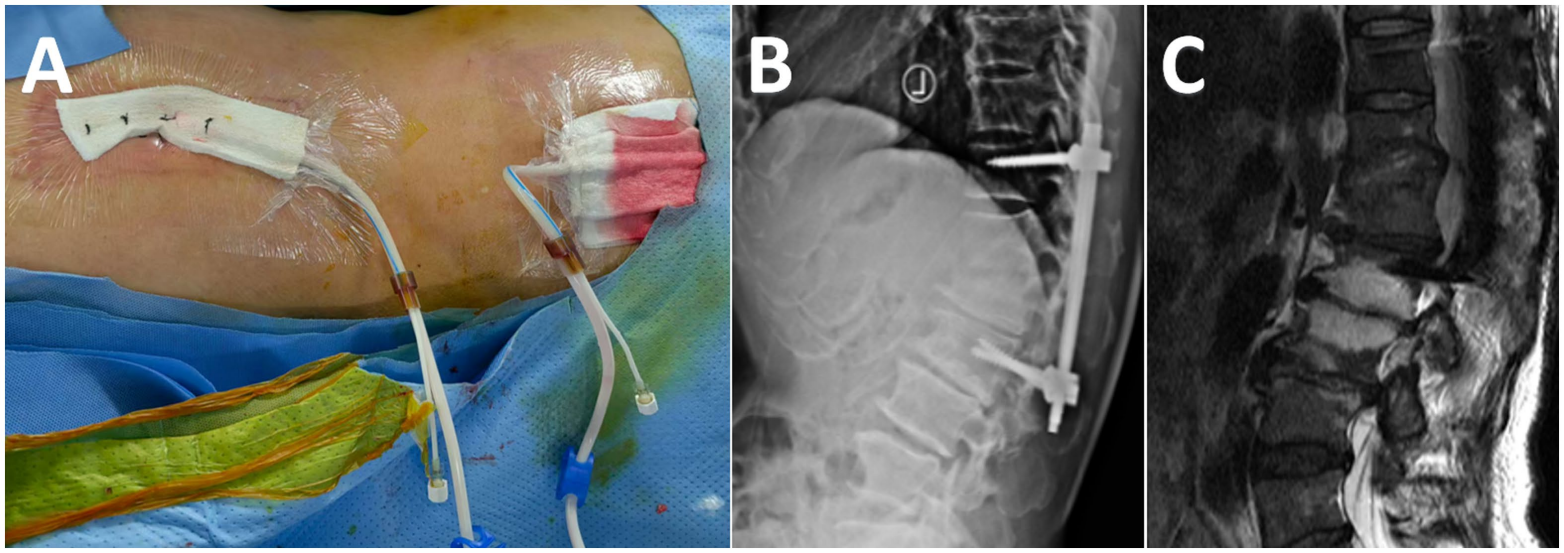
(A) Shows the appearance of postoperative dual-zone VSD drainage. (B) Indicates good fixation after surgery. (C) Shows the disappearance of the intervertebral abscess and inflammatory signal in the vertebral body on MRI 1 month after surgery.
